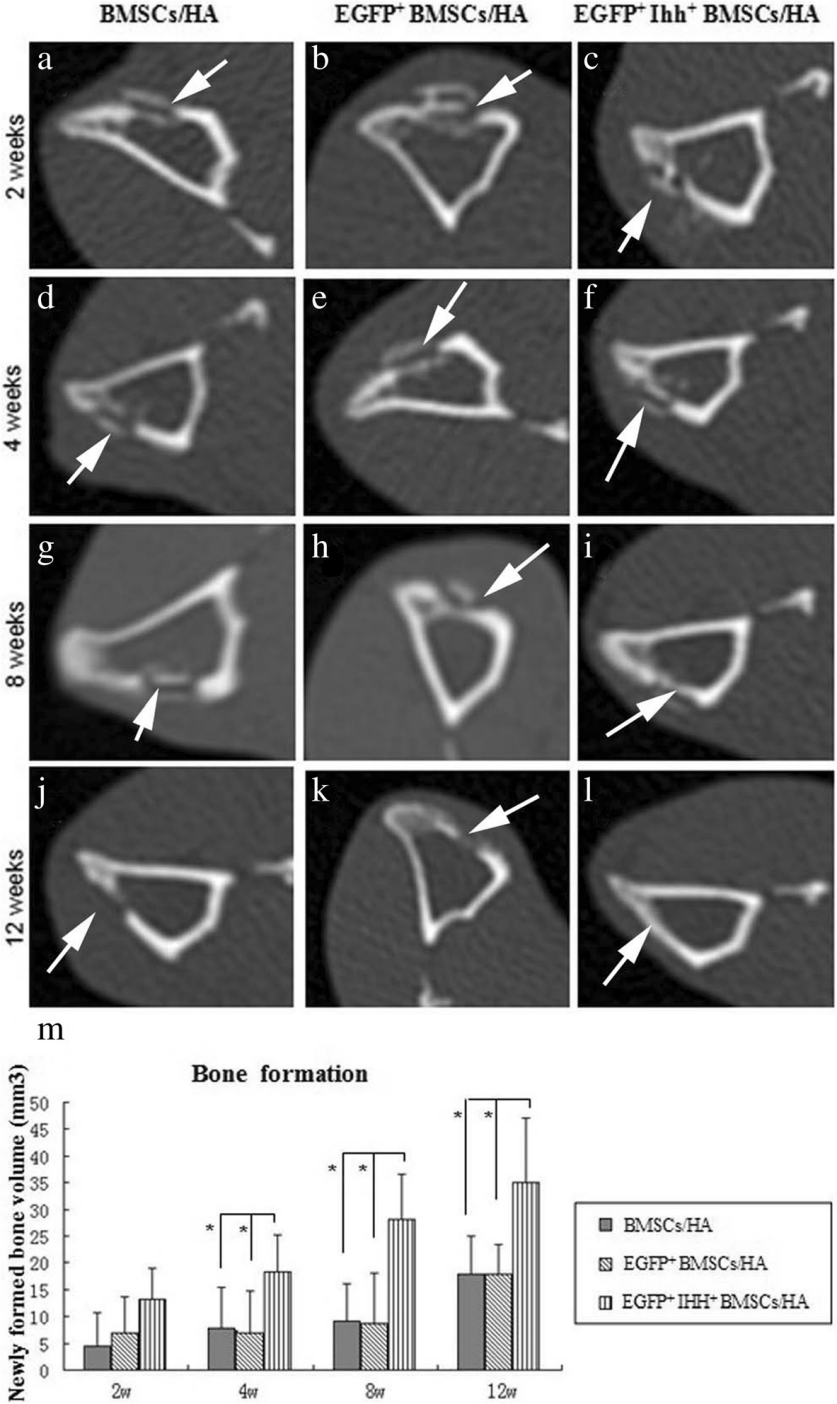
CT images demonstrated that Ihh
